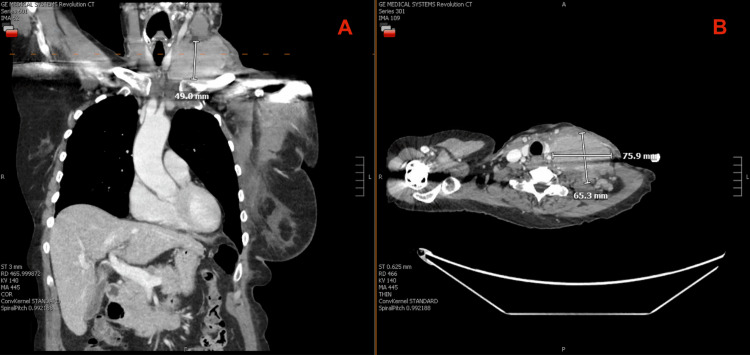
Contrast-enhanced CT of the chest obtained in the ED on October 17, 2024. Coronal (A) and axial (B) CT images demonstrate rapid interval enlargement of the left supraclavicular mass to approximately 76 × 65 mm with vascularity and compression and occlusion of the left internal jugular and subclavian veins, consistent with venous outflow obstruction due to extrinsic tumor compression.
